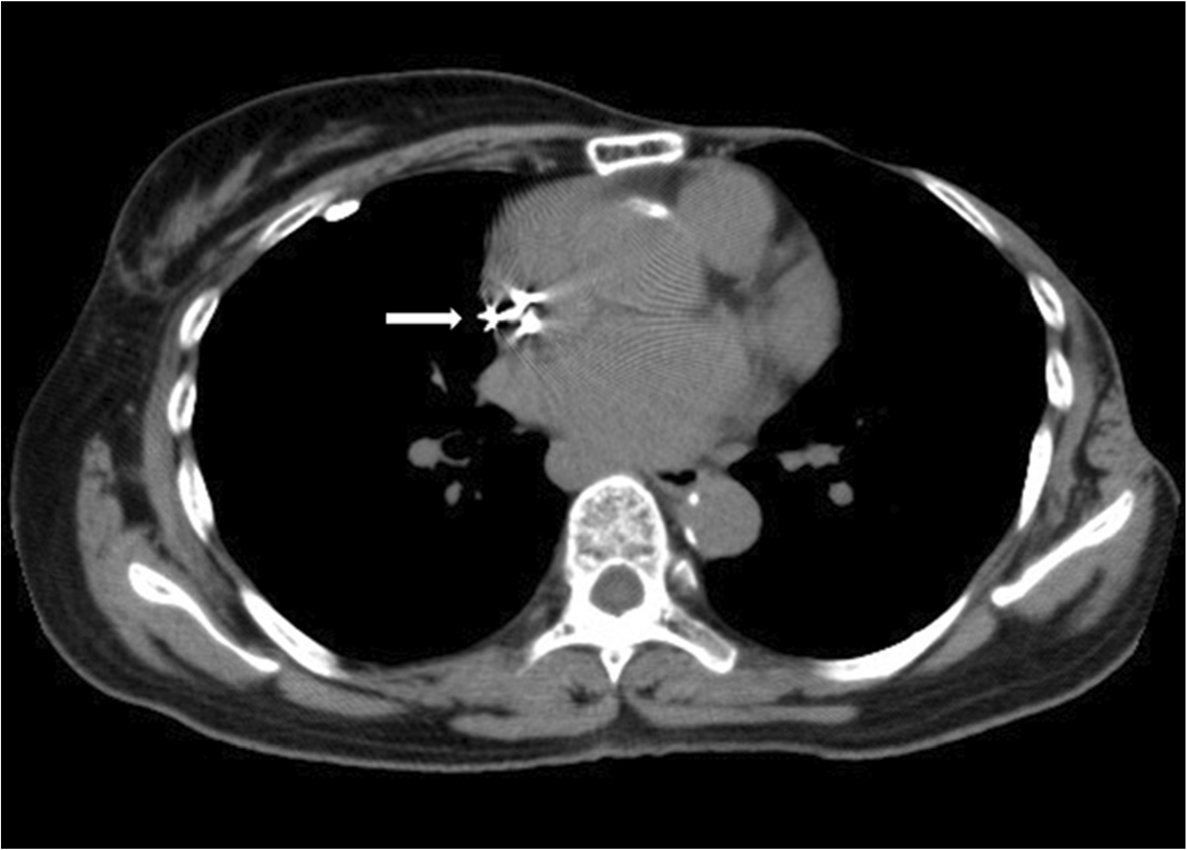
Preoperative chest computed tomography. The atrial lead tip (arrow) was placed in the high lateral part of the right atrium
